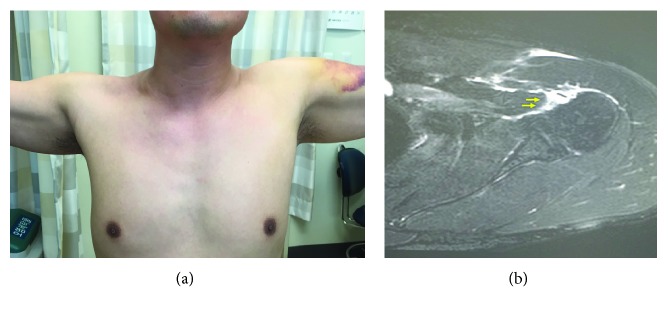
(a) The left shoulder presented with loss of axilla fold contour and bruises over the upper arm. (b) Total rupture of the pectoralis major at humeral insertion showed at T2-weighted axial MRI of the left shoulder.
